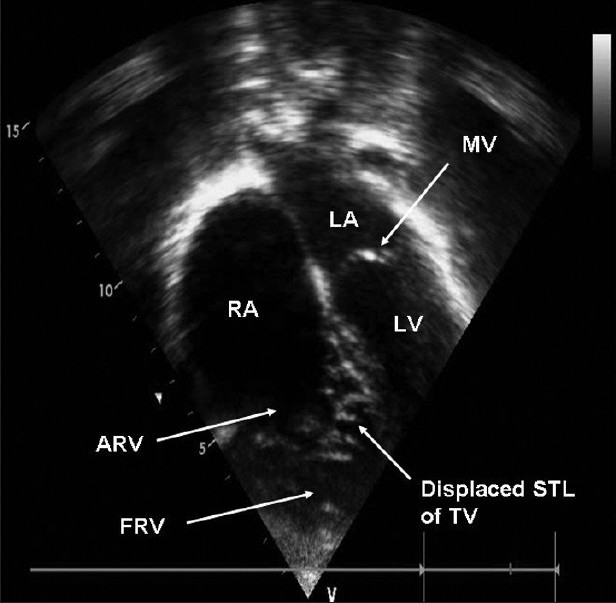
A transthoracic echocardiographic image (at apical four-chamber window) showing apical displacement of the tricuspid valve and atrialized portion of the right ventricle, which is characteristic of Ebstein’s anomaly of the tricuspid valve. (ARV - Atrialized right ventricle, FRV - Functional right ventricle, LA - Left atrium, LV - Left ventricle, MV - Mitral valve, RA - Right atrium, STL - Septal tricuspid leaflet)
